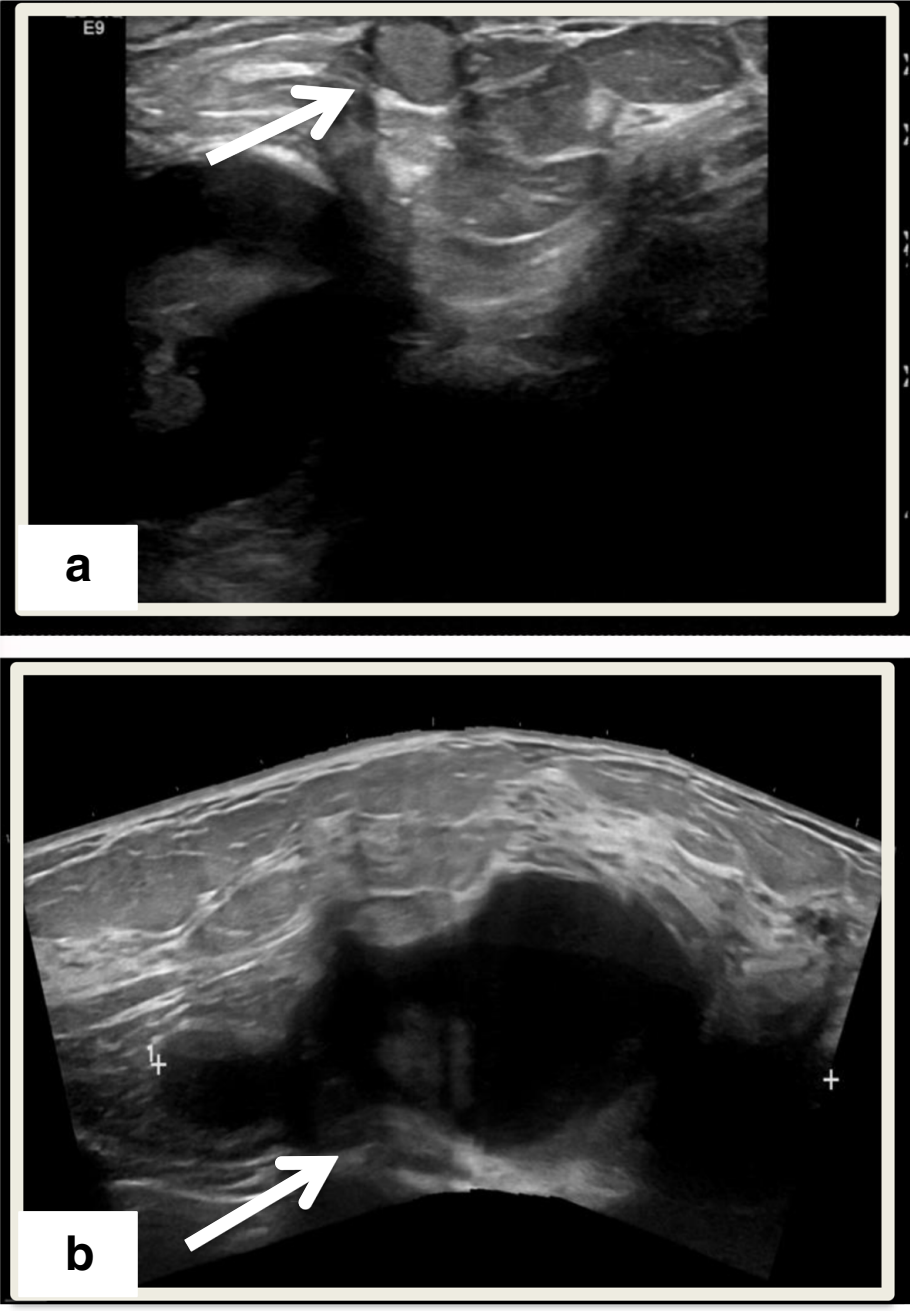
a Ultrasound image of 8-mm solid superficial mass located at six o’clock (white arrow). b Ultrasound image of 7-cm complex cystic mass located at one to three o’clock (white arrow)
